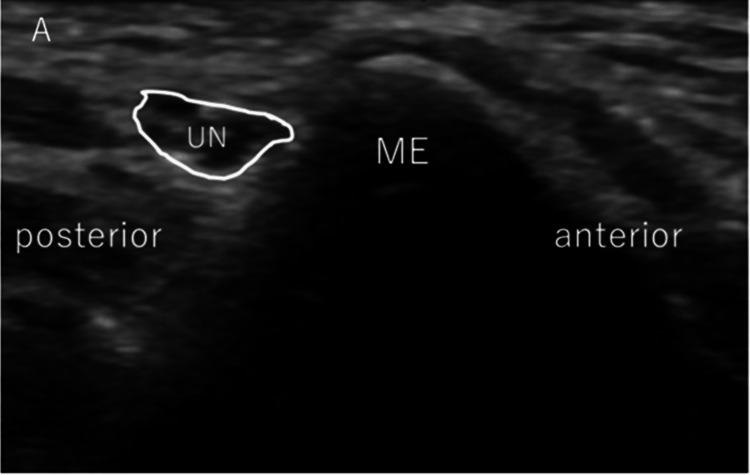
Assessment of ulnar nerve instability: normal type Transverse US images of the ulnar nerve show normal findings. ME: medial epicondyle; UN: ulnar nerve; US: ultrasonography
